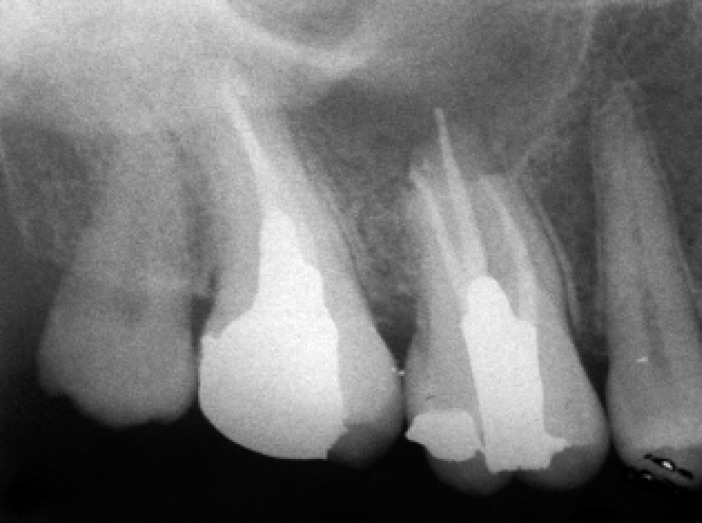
Periapical radio-graph after root canal filling and permanent restoration
